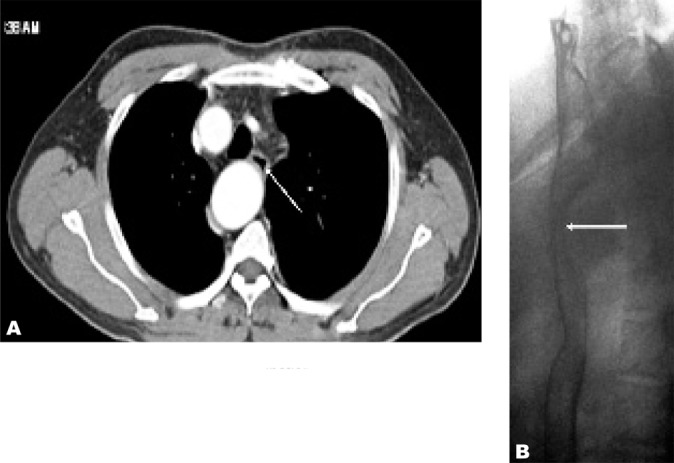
A. Axial CT image shows esophageal compression (arrow) B. Lateral view of barium swallow examination reveals smooth posterior esophageal compression by the aortic arch (arrow).
